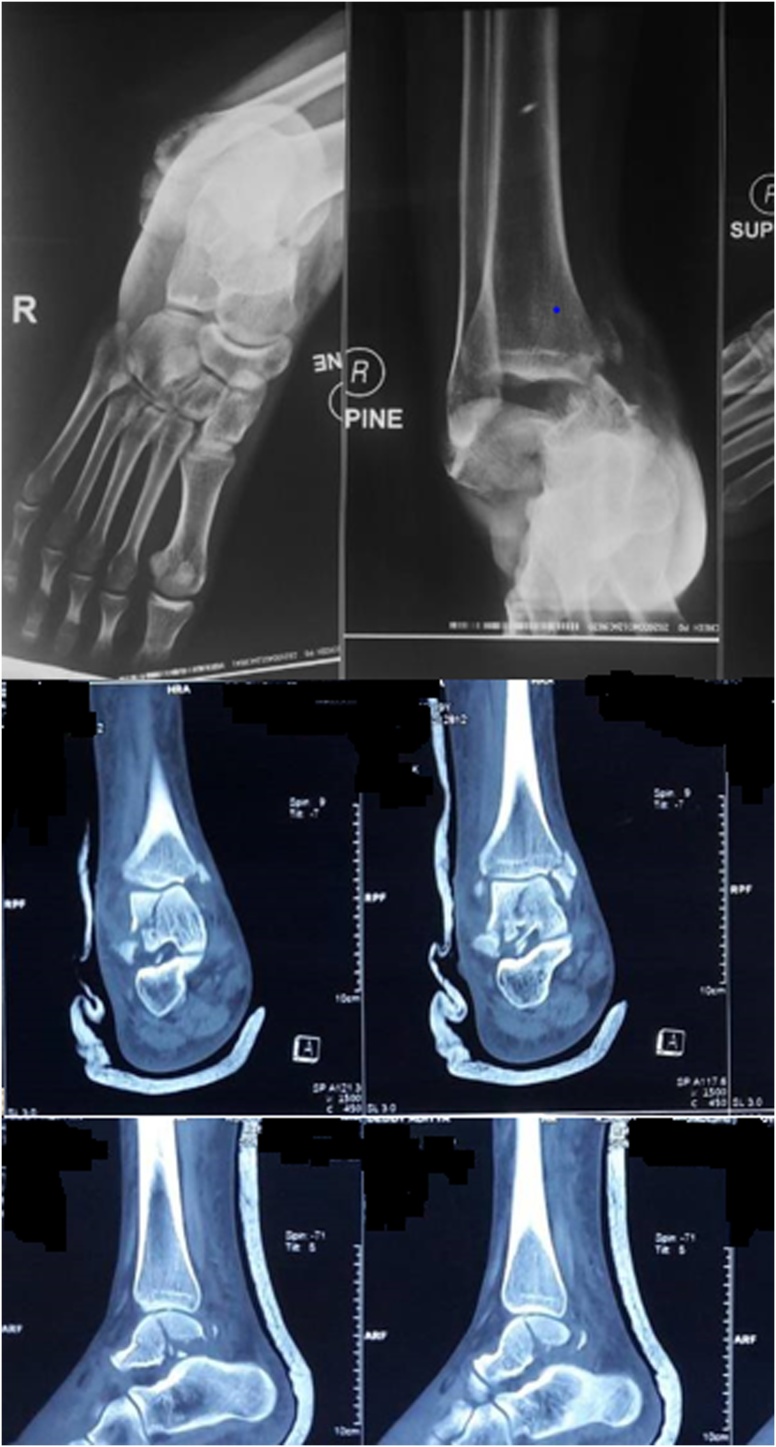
Right Ankle AP Lateral X-Rays and CT Scan showed fracture medial malleolus.
